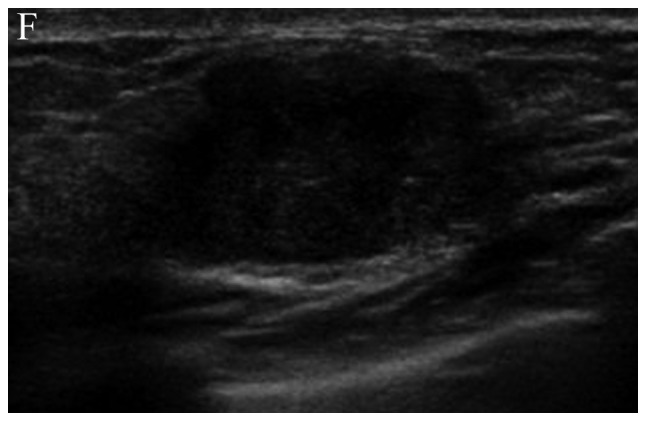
A 40-year-old woman with breast cancer. The breast lesion was enhanced on dynamic MRI before NACT (A) and after final administration of NACT (B). (A and B) The tumor response rate was calculated as 40% by dynamic MRI. A radiologist traced the lesion margin to place the region of interest and calculated the ADC before NACT (C) and after the first cycle of NACT (D). The initial ADC value was 0.879×10^−3^ mm^2^/s, and the %ADC was 14.8% after the first cycle of NACT. Gray-scale ultrasound depicts a hypo-echoic lesion with irregular margins before NACT (E) and after the first cycle of NACT (F). The response rate after the first NACT administration was calculated as −9.5%. NACT, neoadjuvant chemotherapy; ADC, apparent diffusion coefficient.
